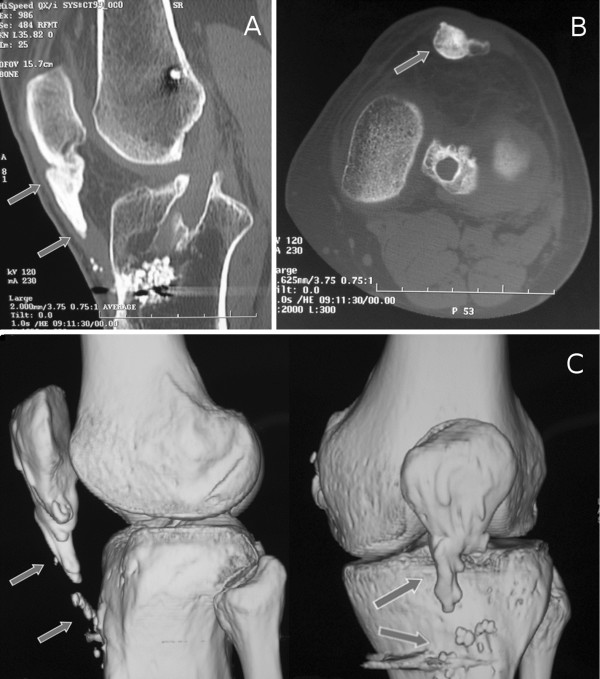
Computed Tomography (CT) scan image of the patient’s left knee at the time of presentation. (A) Sagittal CT scan image shows a 2.8 × 1.2 cm conical ossification of the proximal patellar tendon (arrows). It is present hyperdense material within the tibial tunnel attributable to a resorbable screw. (B) Transverse CT scan image at the joint space level shows patellar tendon ossification (1.5 × 1.2 cm) at the patellar tip (arrows). (C) CT 3D reconstruction images show the proximal and the distal patellar tendon ossification morphology (arrows).
